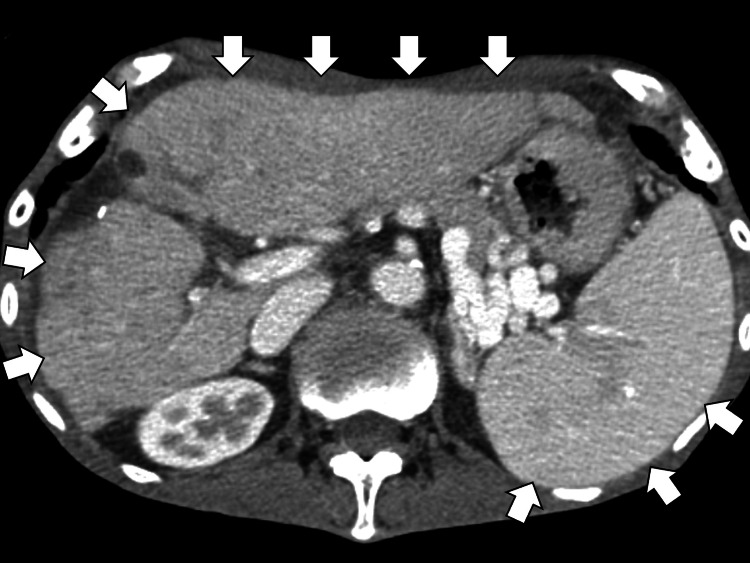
Contrast-enhanced abdominal computed tomography demonstrating splenomegaly in the setting of decompensated liver cirrhosis, suggesting hypersplenism as a potential cause of pancytopenia (white arrows).
